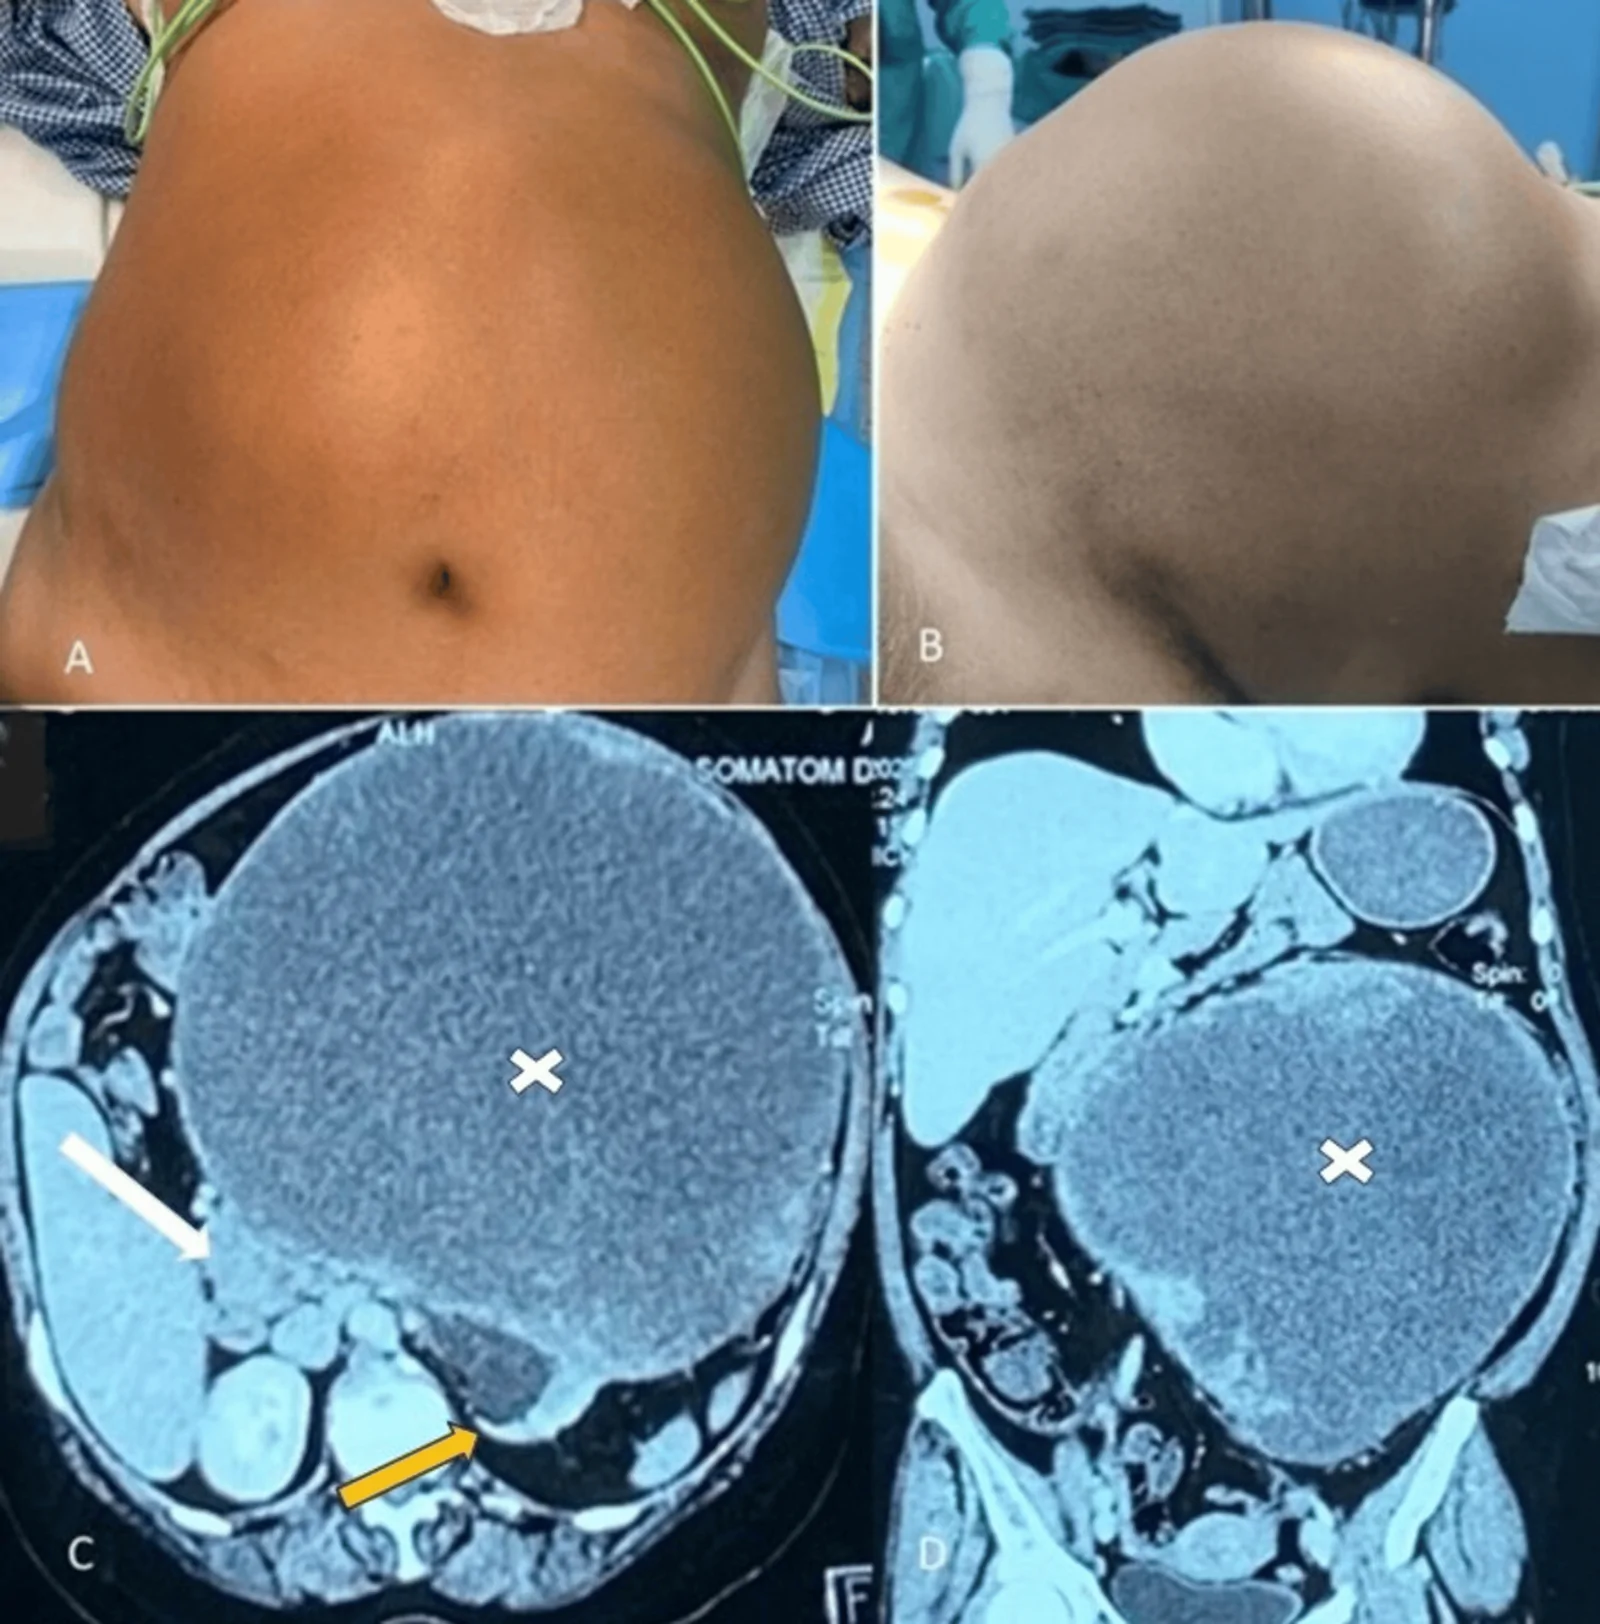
A, B) Preoperative abdominal photographs demonstrating the large abdominal mass; C) axial and D) coronal CECT images demonstrating the giant renal mass (white crosses). The yellow arrow indicates the left kidney, from which the mass originates, and the white arrow indicates displacement of the adjacent abdominal viscera CECT: contrast-enhanced computed tomography
